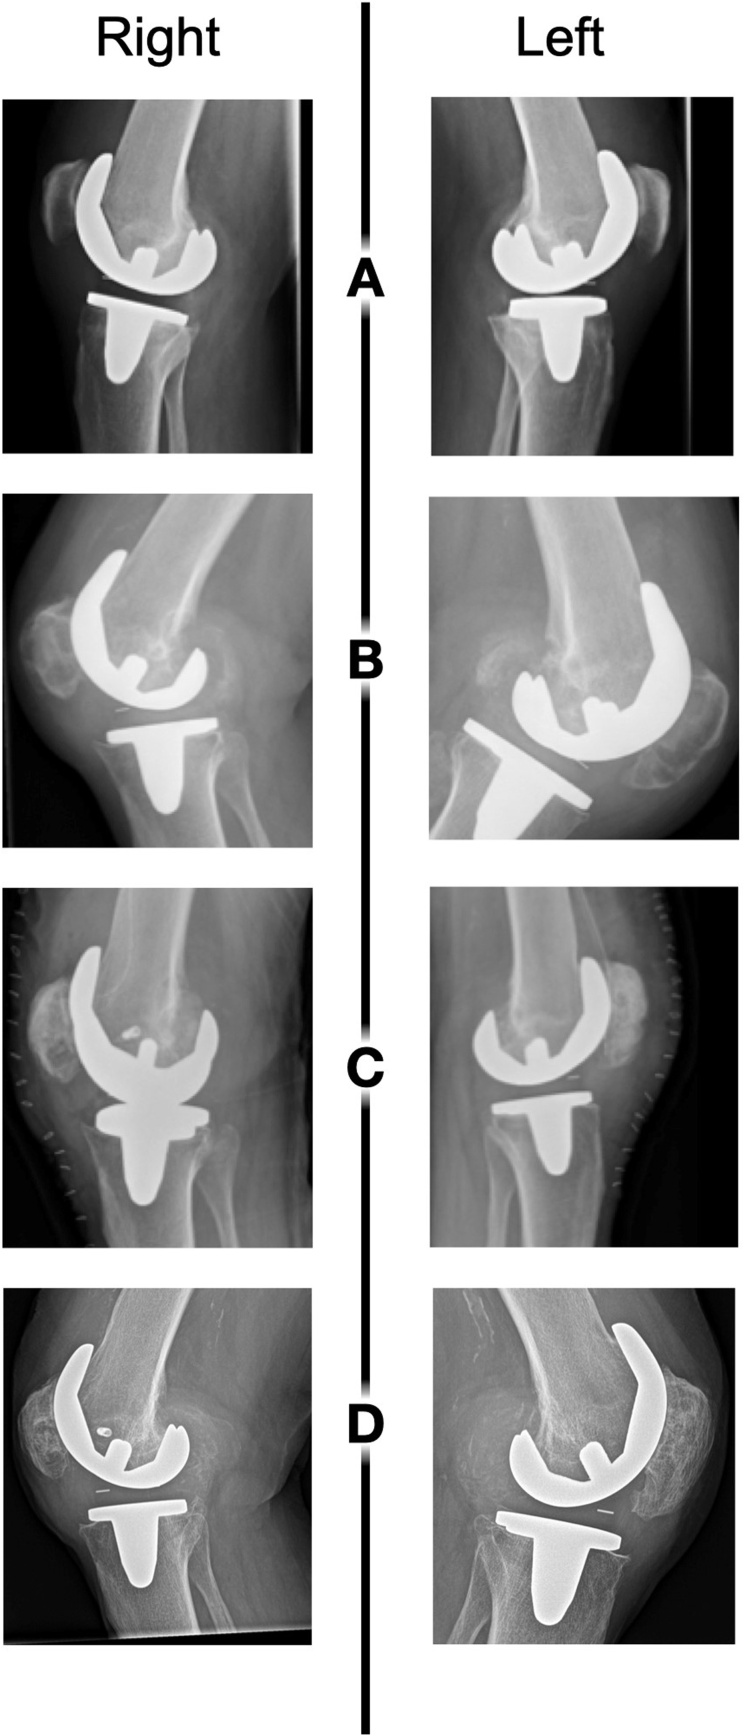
Radiographic diagnosis and follow-up of the massive osteolytic lesions. (A) radiographs after initial index TKA surgery, demonstrating acceptable alignment and no preexistent bone deficiencies; (B) 4 years post index TKA surgery the radiographs showed massive osteolytic lesions in the posterior aspect of the femur and center of the patella in both knees; (C) directly after revision, with allograft bone impaction grafting and collateral ligament fixation (right) visible; (D) 8 years post revision and treatment of the osteolysis, showing no relapse or development of new lesions.
